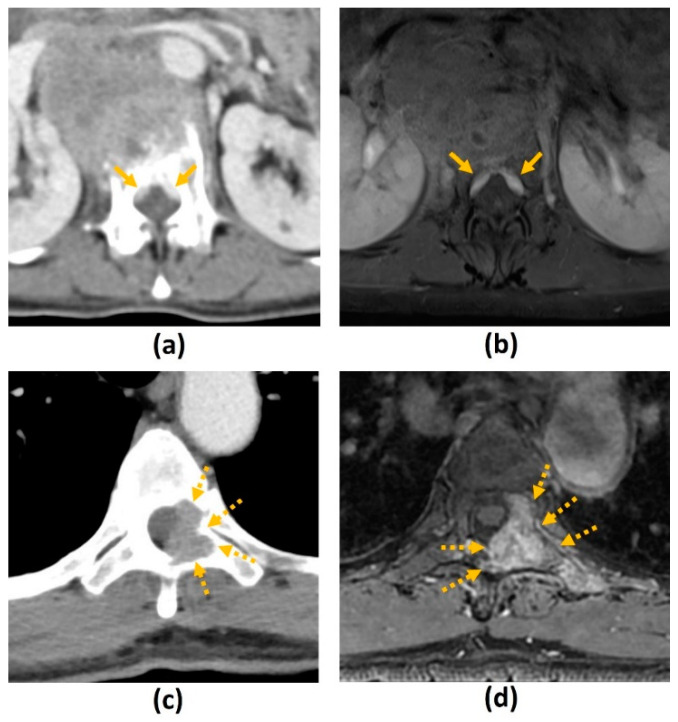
A 51-year-old rectal cancer patient with spine metastasis and concurrent inferior vena cava obstruction with resultant dilatation of the epidural venous plexus. Axial CT images in the soft tissue window (a), and axial post-contrast fat-suppressed T1-weighted (b) MR sequences of the spine. The dilated epidural veins (solid arrows) mimic an enhancing soft-tissue lesion in the epidural space and can be mistaken for metastatic spinal cord compression (MSCC). Another 68-year-old lung cancer patient with spine metastasis. Axial CT images in the soft tissue window (c), and axial post-contrast fat-suppressed T1-weighted (d) MR sequences of the spine. Enhancing epidural disease (dotted arrows) causes high-grade (Bilsky 2) MSCC.
